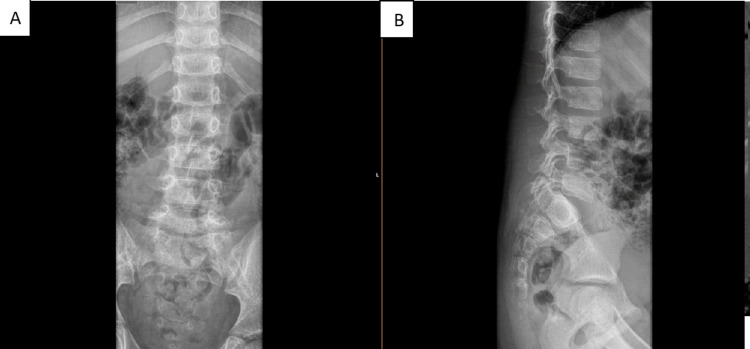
Radiograph after removal of the osteosynthesis material (A) Anteroposterior view. (B) Lateral view
